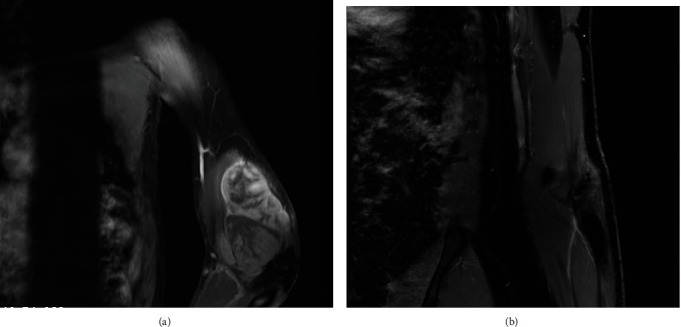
(a) This MRI image was taken at first presentation in 2011. It showed a large lobulated heterogeneous mass extending from the middle of the left arm down to the middle of forearm, measuring 29 × 9 × 9 cm. (b) The last MRI imaging done dated on January 2018. No evidence of circumscribed mass lesion or abnormal enhancement to suggest tumor recurrence. No new lesions identified.
